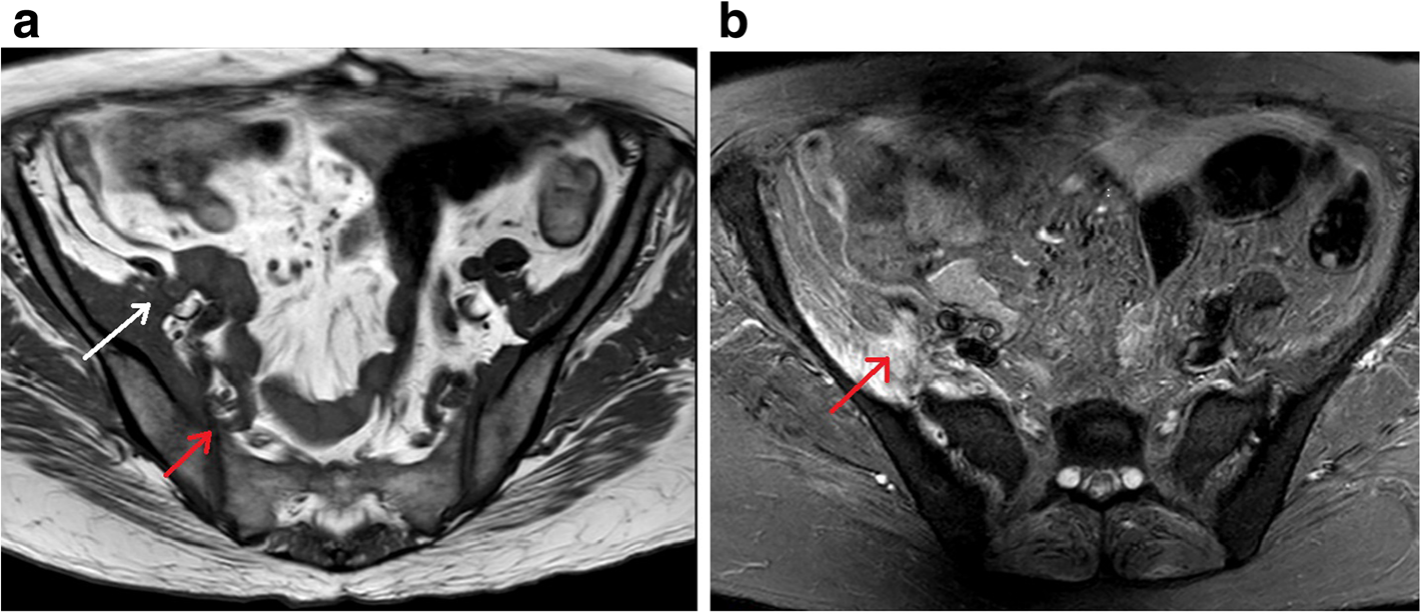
Coronal view of MRI sacroiliac joints: (during intensive phase treatment) a T1 Weighted Image showing thickening of right internal iliacus muscle(white arrow) and erosion of anterior joint space of right iliac joint(red arrow), b Fat supressed (STIR) image showing thickening of right internal iliacus muscle, oedema of fat planes anterior to right iliac bone and sacrum with extension into anterior joint space of right iliac joint(red arrow)
